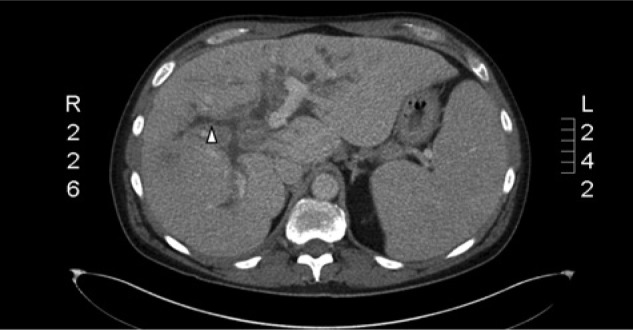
Liver computed tomography after drain placement. While dilated intrahepatic biliary ducts (white arrow head) persist throughout the liver, the liver abscess is no longer visible.
